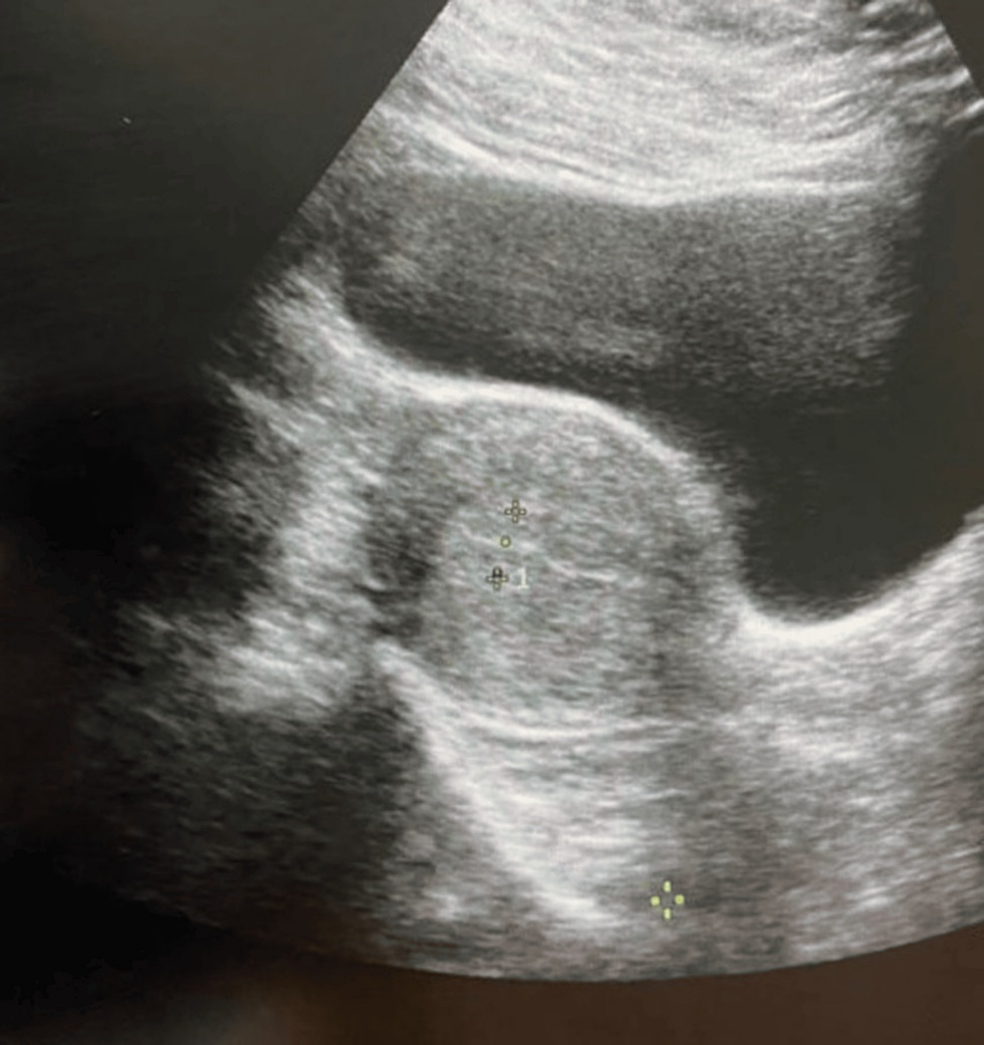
Ultrasonography of the pelvis with normal findings of uterus and no signs of intraperitoneal hemorrhage.
